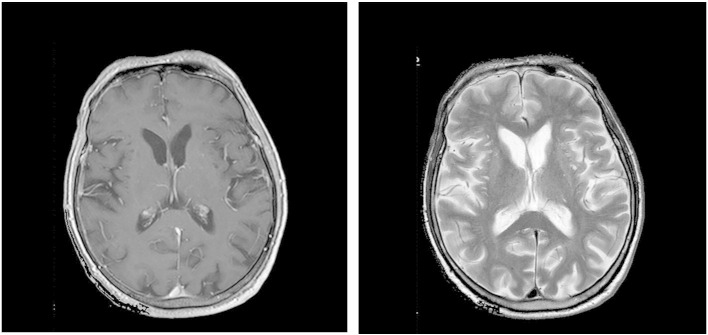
Proband's T1- (left panel) and T2-weighted axial (right panel) MRI at time of presentation without major abnormalities.
